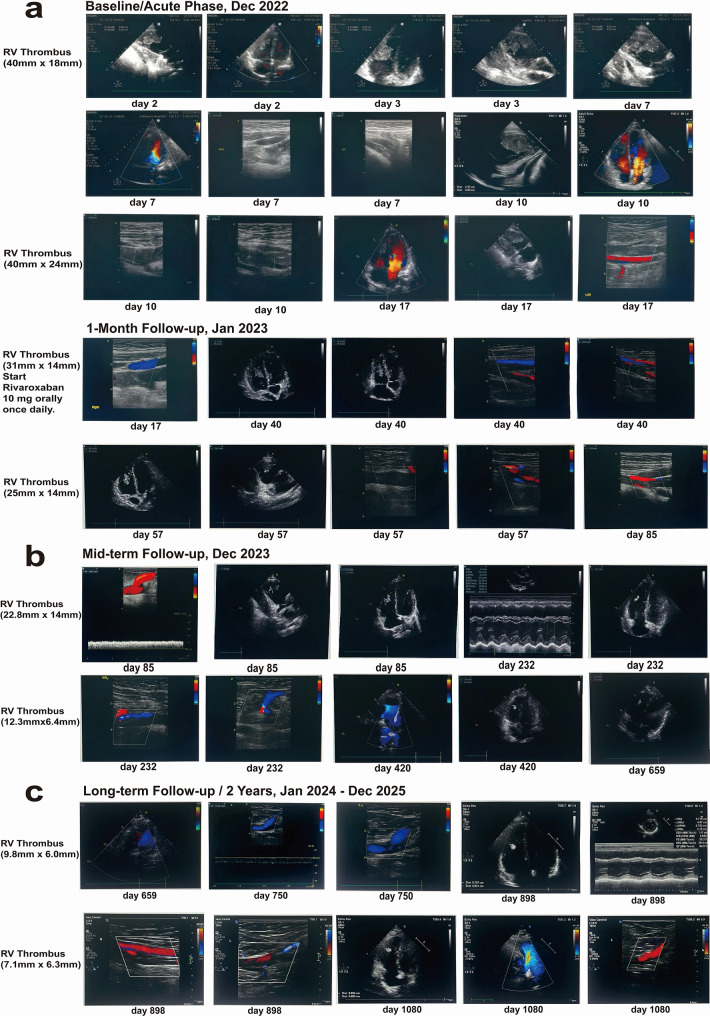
Timeline of echocardiography and CDFI changes throughout disease course and treatment. (a) Trends of echocardiogram and CDFI in children admitted for the first time within 0–43 days, with the patient starting rivaroxaban 10 mg once daily on day 40. (b) During the middle stage of treatment, subsequent regression of the thrombus. (c) During a 2-year follow-up in the long-term treatment process, echocardiography showed regression of thrombus size
